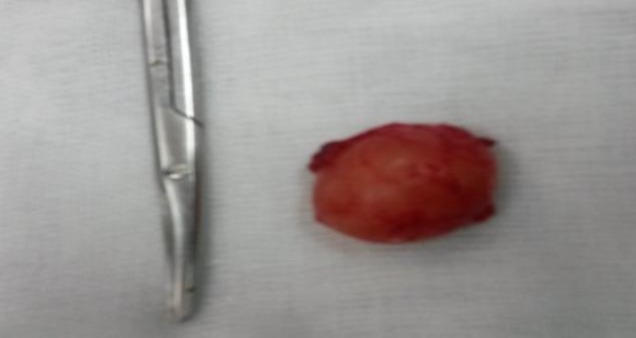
CT scan of sinus revealing a thickness in the right maxillary sinus and a defect in the anterior aspect of the nasal septum
